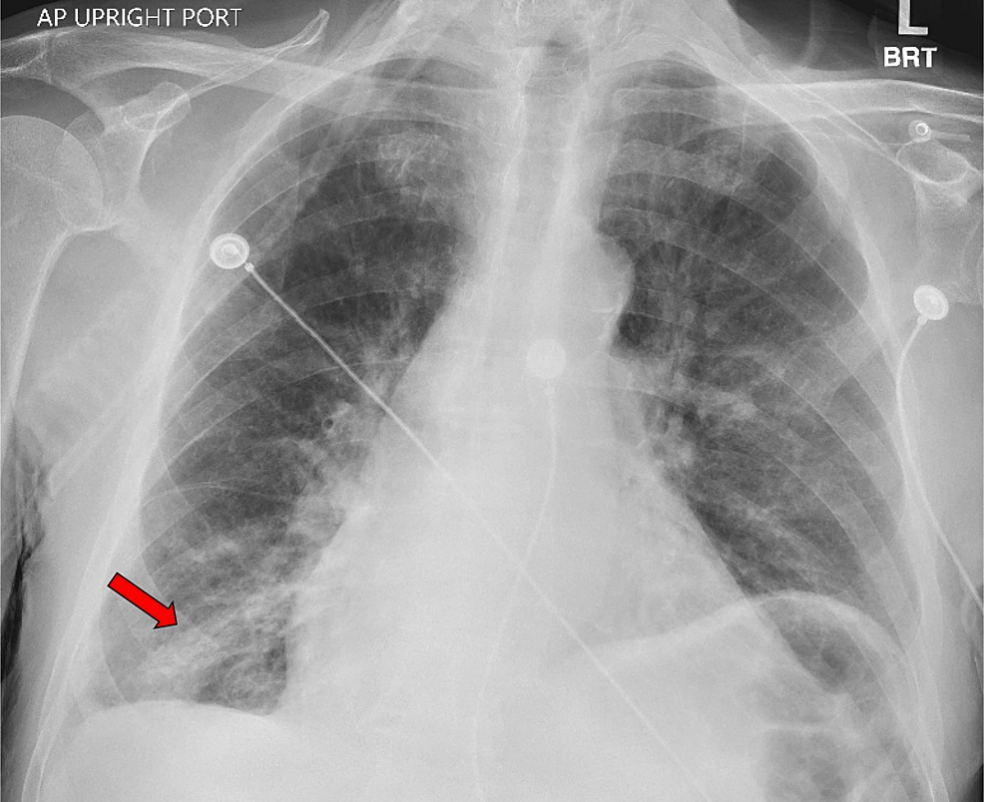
Patient’s initial AP chest X-ray taken in the emergency room A right lower lobe infiltrate can be seen (arrow) consistent with pneumonia and signs of congestive changes. AP: anteroposterior
